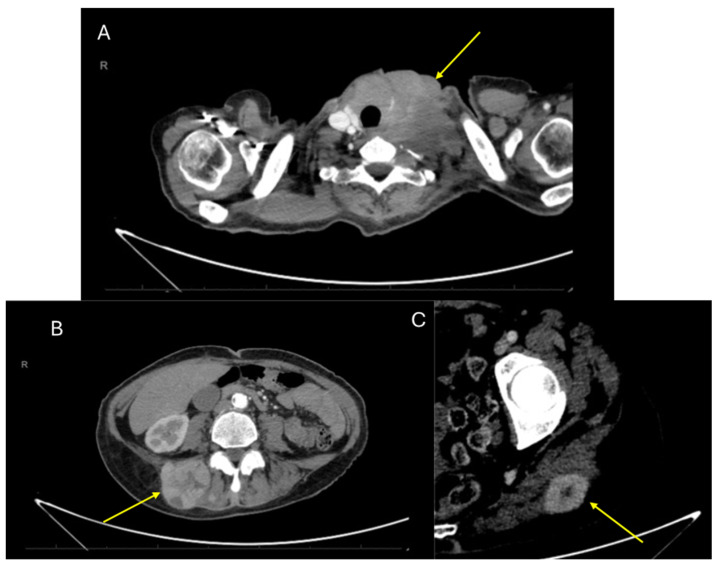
CT images of recurrences during the follow-up period: (A) Extensive supraclavicular recurrence 4 years after radical surgery. Absence of the vascular bundle of the neck, resected in radical cervical dissection surgery. (B) Contralateral paravertebral metastasis 3 years after diagnosis. (C) Ipsilateral gluteal metastasis 3 years after initial diagnosis.
